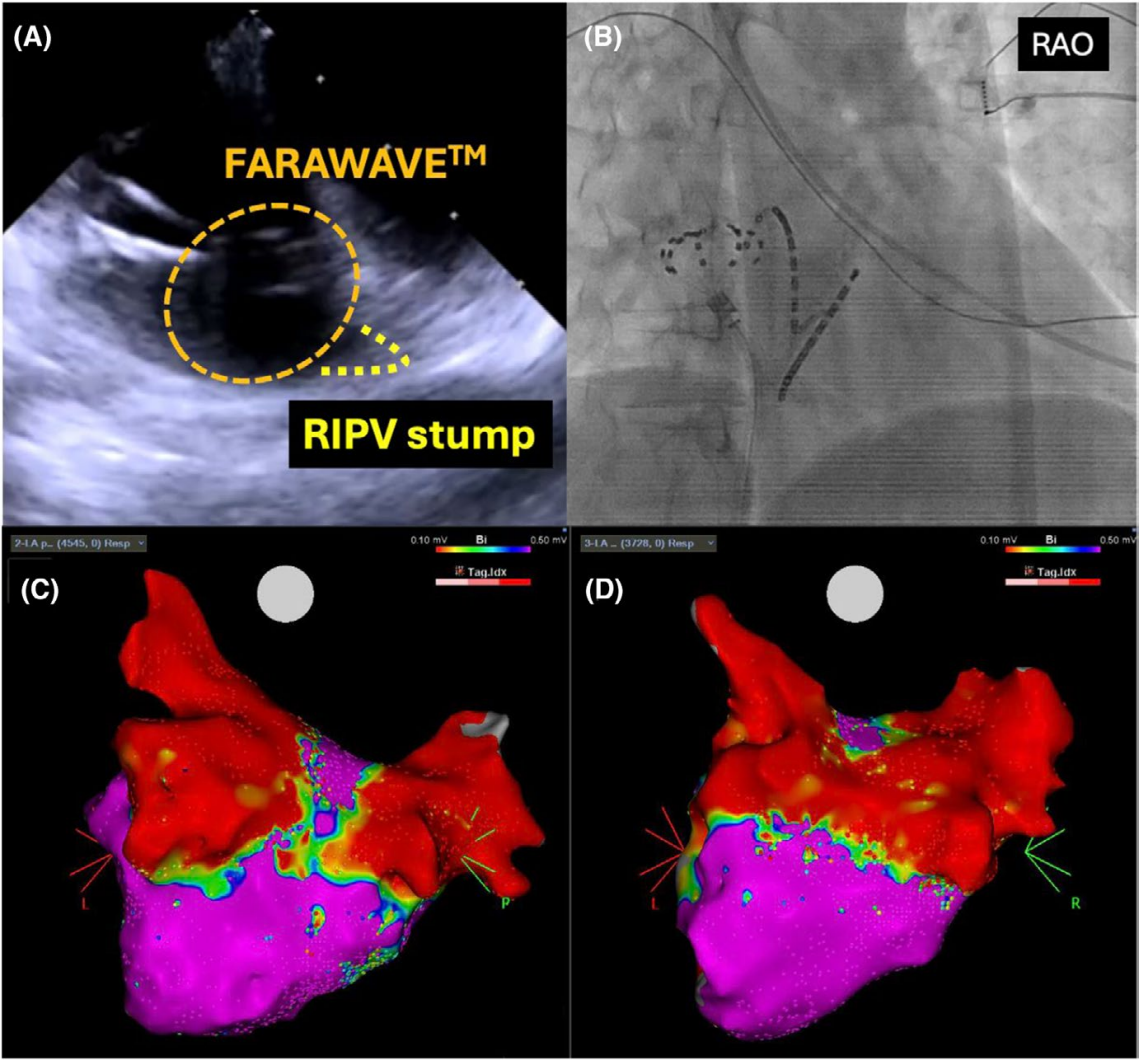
(A) Intracardiac echocardiography (ICE) confirmed that the pentaspline catheter (FARAWAVE™) was in contact with the right inferior pulmonary vein (PV) antrum. (B) The fluoroscopic image shows that the FARAWAVE™ catheter in a basket configuration is positioned at the RIPV in the RAO view. (C) Voltage map of the left atrium (LA) after the PVI obtained with OCTARAY™ (PA view). The 3D‐EAM revealed that the left atrial posterior wall was nearly isolated and demonstrated an unexpected isthmus on the posterior wall. (D) Additional applications rendered the inferior line of the left atrial posterior wall.
